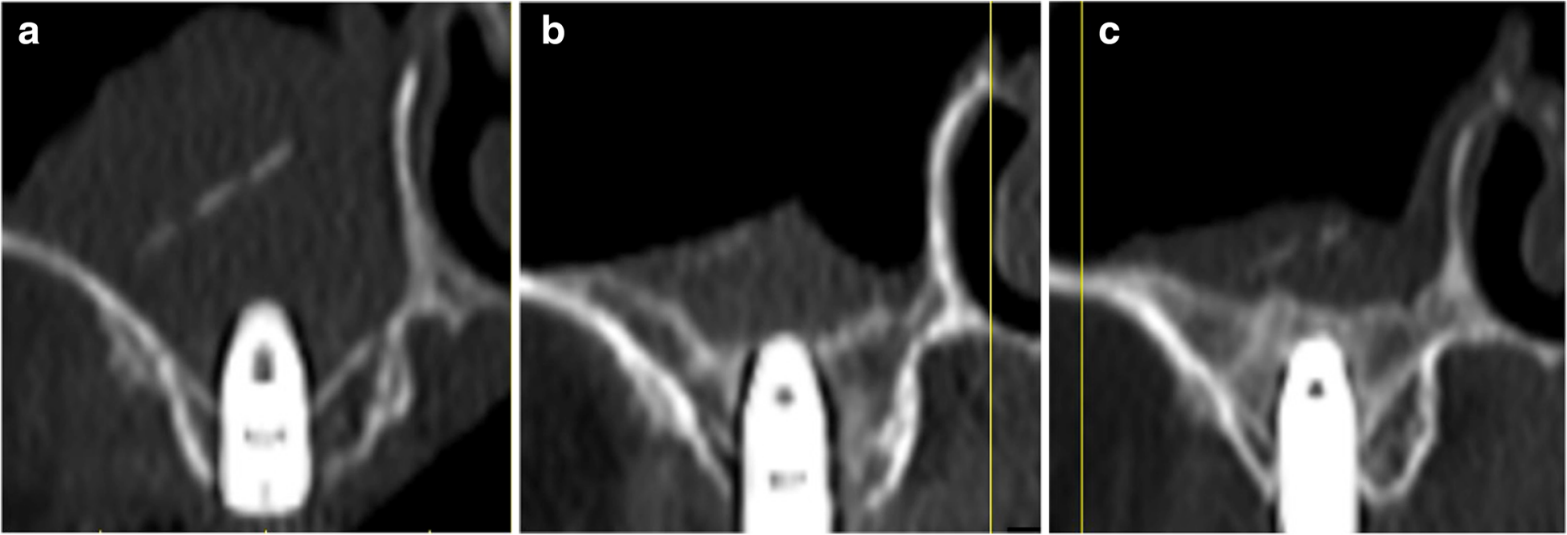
Radiographic findings on cross-sectional computed tomography in the right second molar region after nongrafted sinus lift with simultaneous implant placement. a Immediately. b At 6 months. c At 42 months
